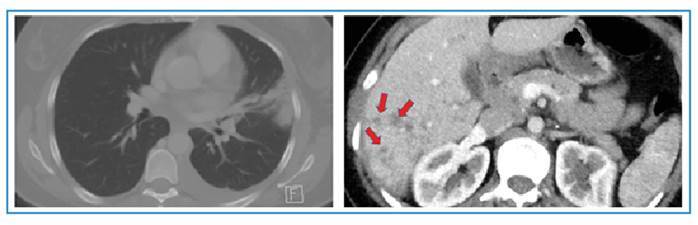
Thoracic computed tomography images showing a consolidated area with air bronchograms in the upper lobe of the left lung, minimal pleural effusions in the lower lobe of the left lung and heterogeneous lesions in the liver (red arrows).
